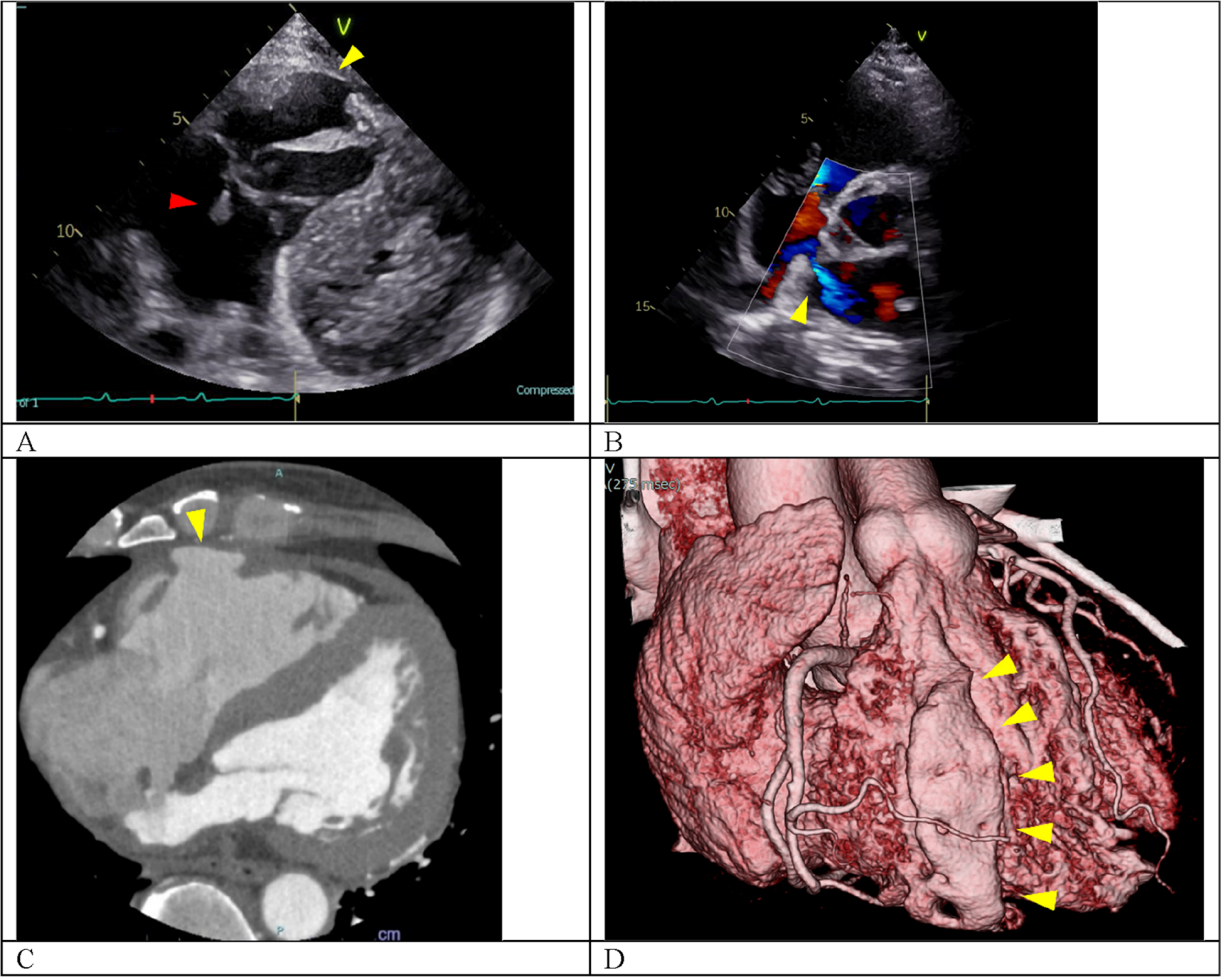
A Preoperative echocardiography reveals tricuspid valve prolapse caused by papillary muscle rupture (red arrow), and a thin area of the right ventricular anterior wall (yellow arrow). B Color Doppler echocardiography detects a bidirectional shunt across the atrial septum (arrow). C Preoperative contrast-enhanced computed tomography shows that the right ventricular anterior wall has a thin region posterior to the sternum (arrow). D Three-dimensional computed tomography reconstruction reveals that the right ventricular anterior wall injury corresponds to the sternum (arrows)
